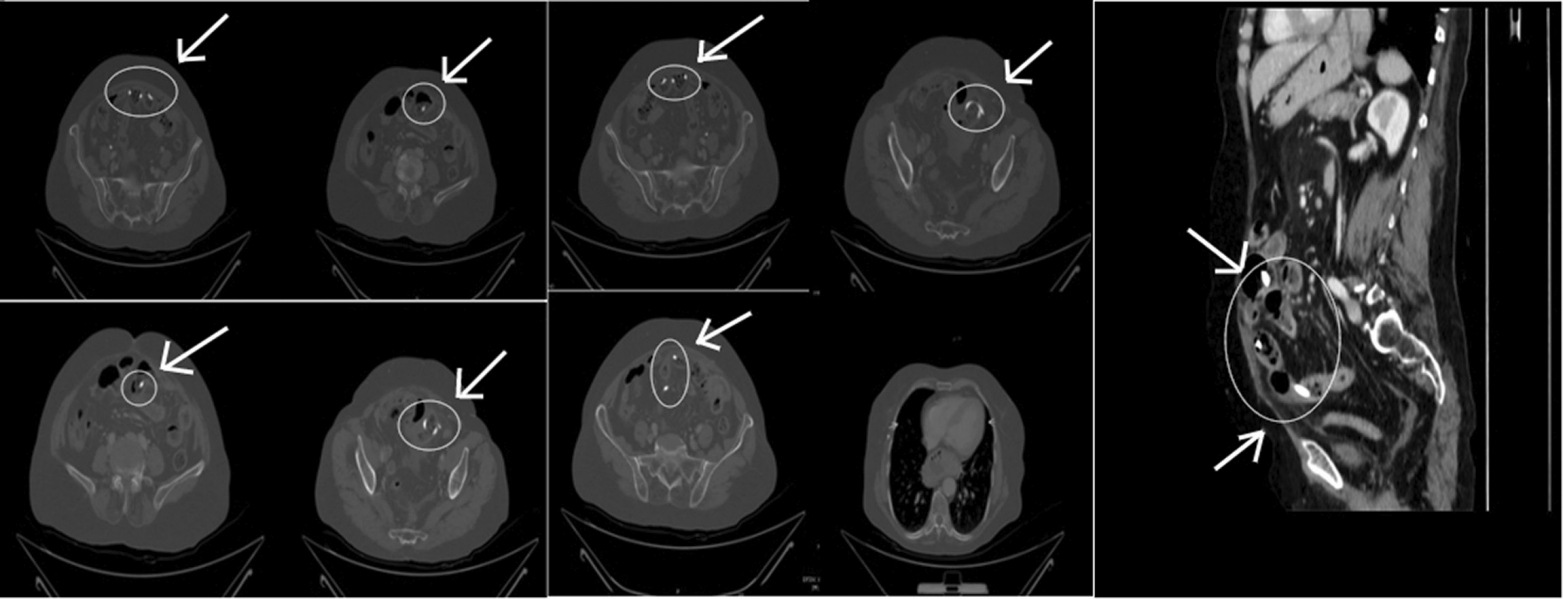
Computed tomography scan; a multiple serial axial and reformatted sagittal images showing the findings.
